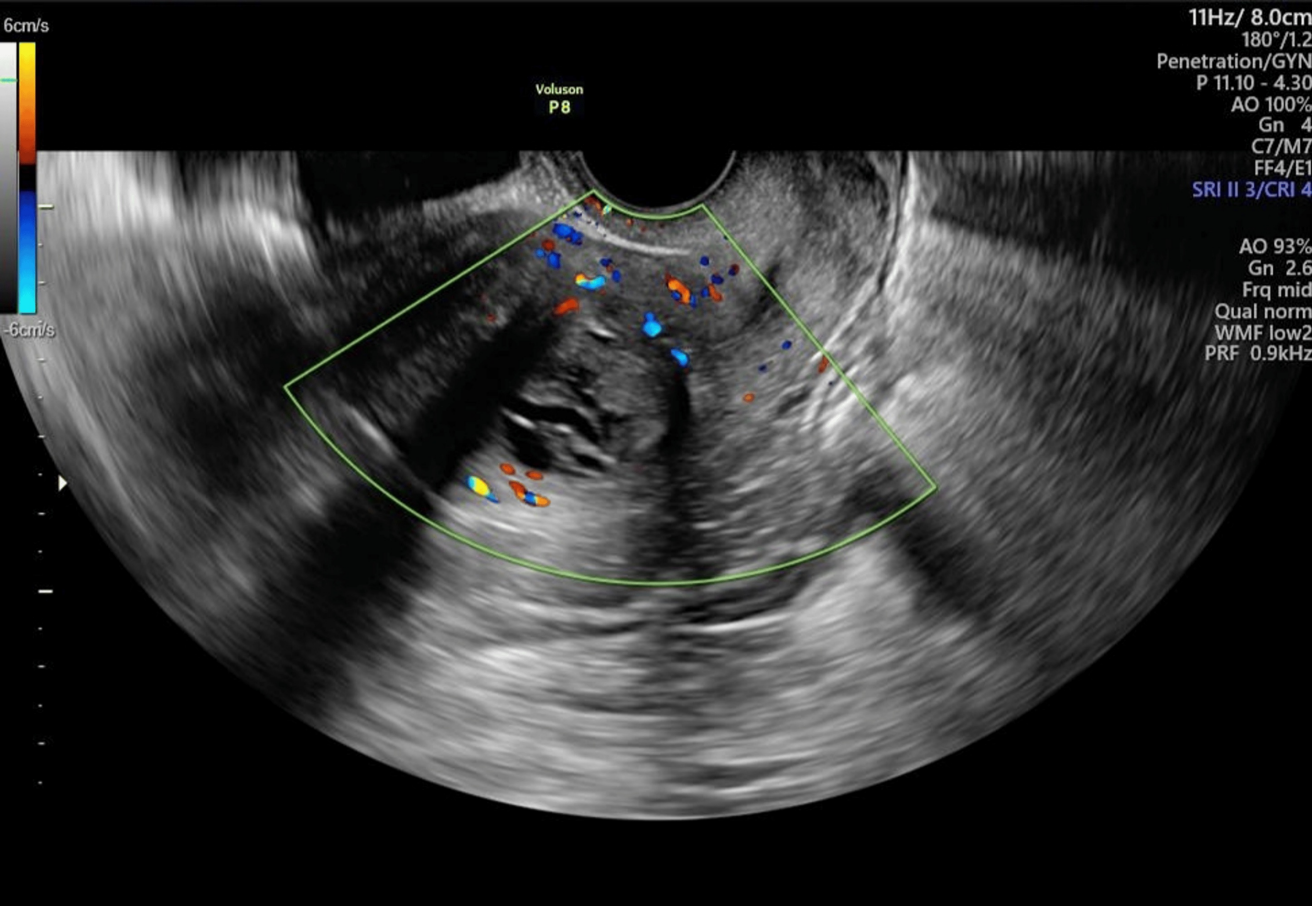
Transvaginal ultrasonography The image shows a 40-mm lesion in the myometrium of the uterine body with calcification and edema. Color Doppler imaging showed blood flow along the margins of the mass.
